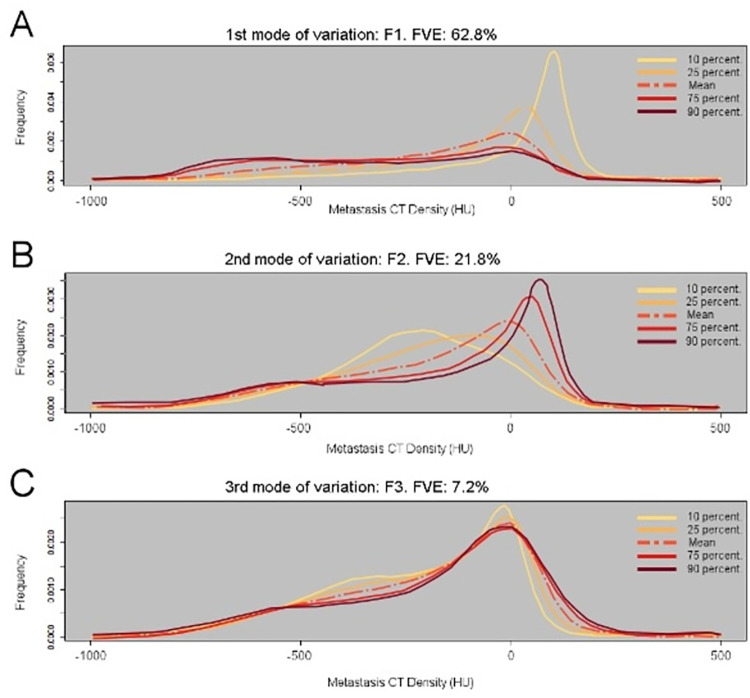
Functional principal component analysis of the metastasis CT density curves. A. First mode of variation (F1). B. Second mode of variation (F2). C. Third mode of variation (F3). FVE: Fraction of the variance explained.
